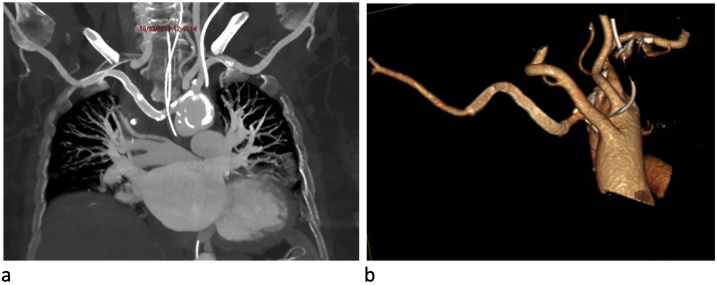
CT scan showing correct positioning of the endovascular stent graft (a); 3D CT reconstruction (b).
